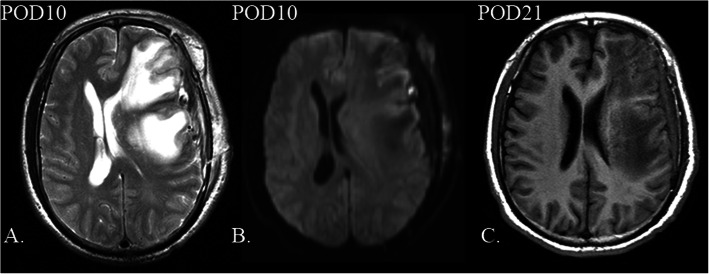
Postoperative magnetic resonance imaging (MRI) findings. a. and b. T2-weighted magnetic resonance imaging (MRI) and diffusion-weighted imaging indicate no infarction occurred on the left side, but massive cerebral edema on the 10th day after surgery. c. T1-weighted MRI showed mild migration of the middle line on the 21st day after surgery
